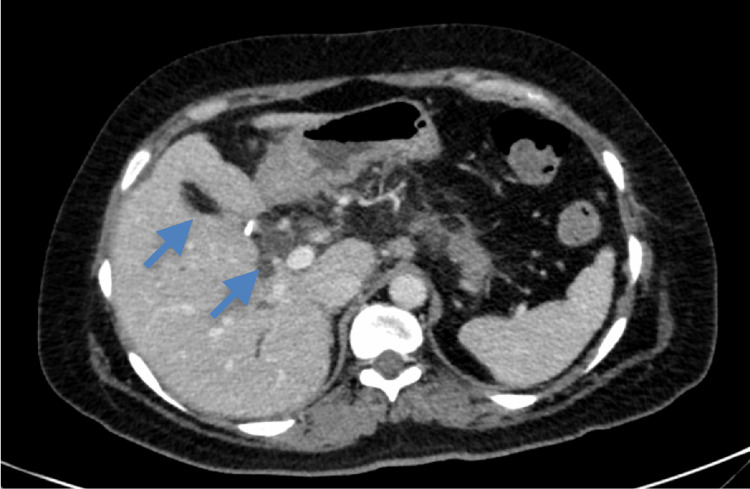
CT scan of the abdomen/pelvis showing biliary duct abnormalities.
